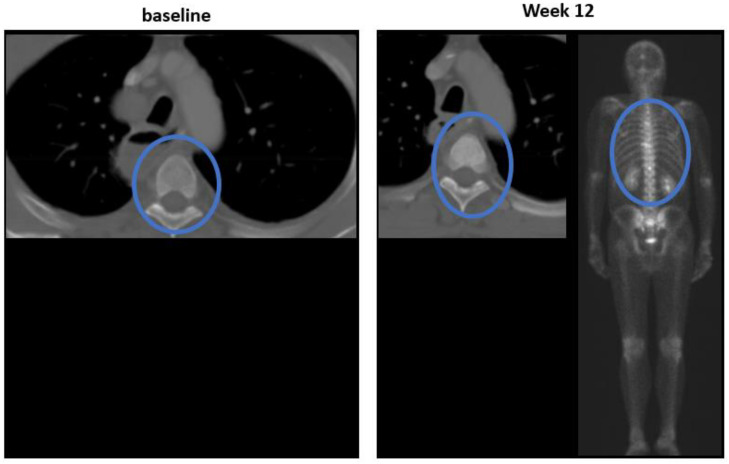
New sclerotic lesions. Newly appearing sclerotic lesions in the CT and uptakes in the bone scan in week 12, but with no evidence of lesions at baseline (marked in blue circles).
